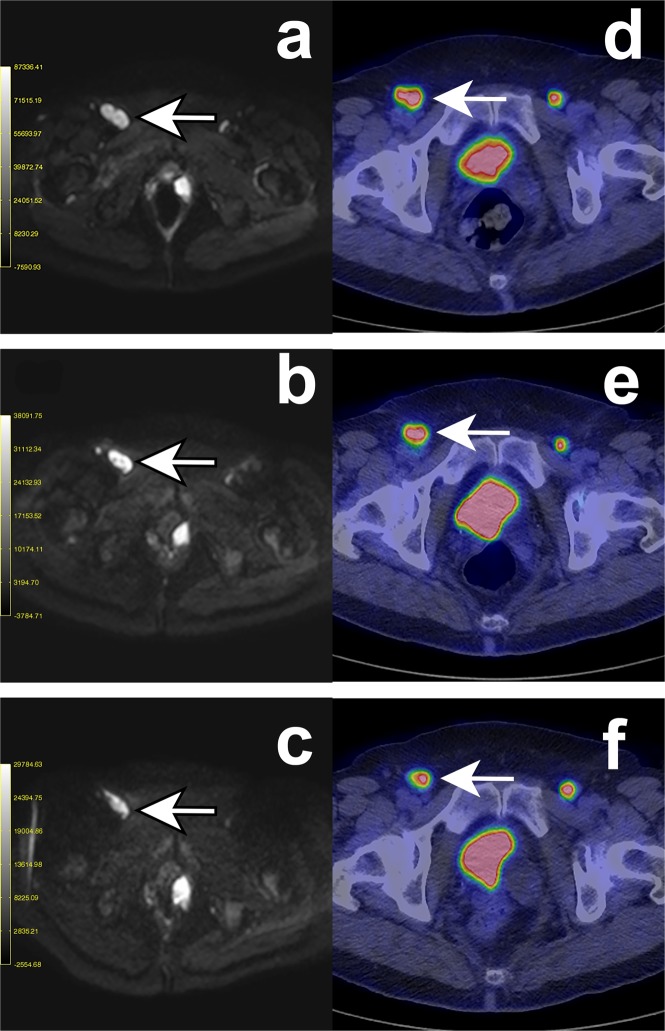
DW- and PET-images of a 67-year-old woman with disseminated mucosal melanoma in vulva (cohort 2). Four intratumoral injections with AdCD40L were given to a right inguinal lymph node metastasis. The patient had partial metabolic response (PMR) in the first and stable metabolic disease (SMD) in the second post-therapy evaluation, according to EORTC criteria while the case was assessed as stable disease (SD) at both post-therapy scans according to RECIST 1.1. An increase in ADC/D in the injected metastasis was observed at both DW-MRI scans post-therapy. The short axis of the injected metastasis was measured at each time date and was unaltered according to the RECIST 1.1 criteria. An initial decrease of the f% value was observed while it was increased at the second DW-MRI scan post-therapy. In a not injected left inguinal lymph node metastasis the ADC-value was increased at both DW-MRI scans post therapy while the value of D was initially increased but it was decreased in the second post-therapy evaluation. A similar pattern as for the D-value was observed regarding the values of f% while the size of the metastasis was unaltered. A third metastasis at the proximity of the urinary bladder is present in the DW-images. This metastasis was not distinguishable at the PET/CT scans due to the high activity of the urinary bladder. (a–c) Diffusion-weighted MR image (DWI) at b = 900 s/mm^2^ -in the axial plane. The arrows indicate the injected right inguinal metastasis at baseline (a), in Scan 1 at week 5 (b) and in Scan 2 at week 9 (c). (d–f) FDG-PET images. The arrows indicate the injected right inguinal metastasis at baseline (a), in Scan 1 at week 5 (b) and in Scan 2 at week 9 (c).
